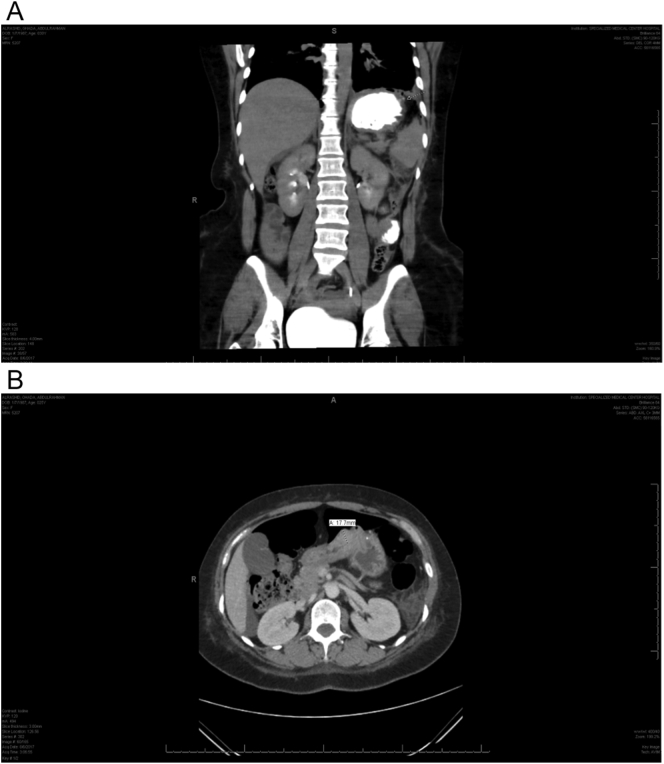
a/b: Abdominal CT with IV and oral contrast showing evidence of a previous surgical procedure along the distal body of the stomach and antrum, with thickening of the antrum wall, and a mildly distended stomach (likely related to the patient's surgical history). The scan also suggests leakage of a track of contrast from the gastric fundus. A large amount of pneumoperitoneum is also seen with intra-abdominal free fluid. Additionally, there is minimal left-sided pleural effusion, with atelectasis of the basal segments of both lower lobes. There is also a small right pleural air bubble, likely caused by air leakage from the abdomen.
